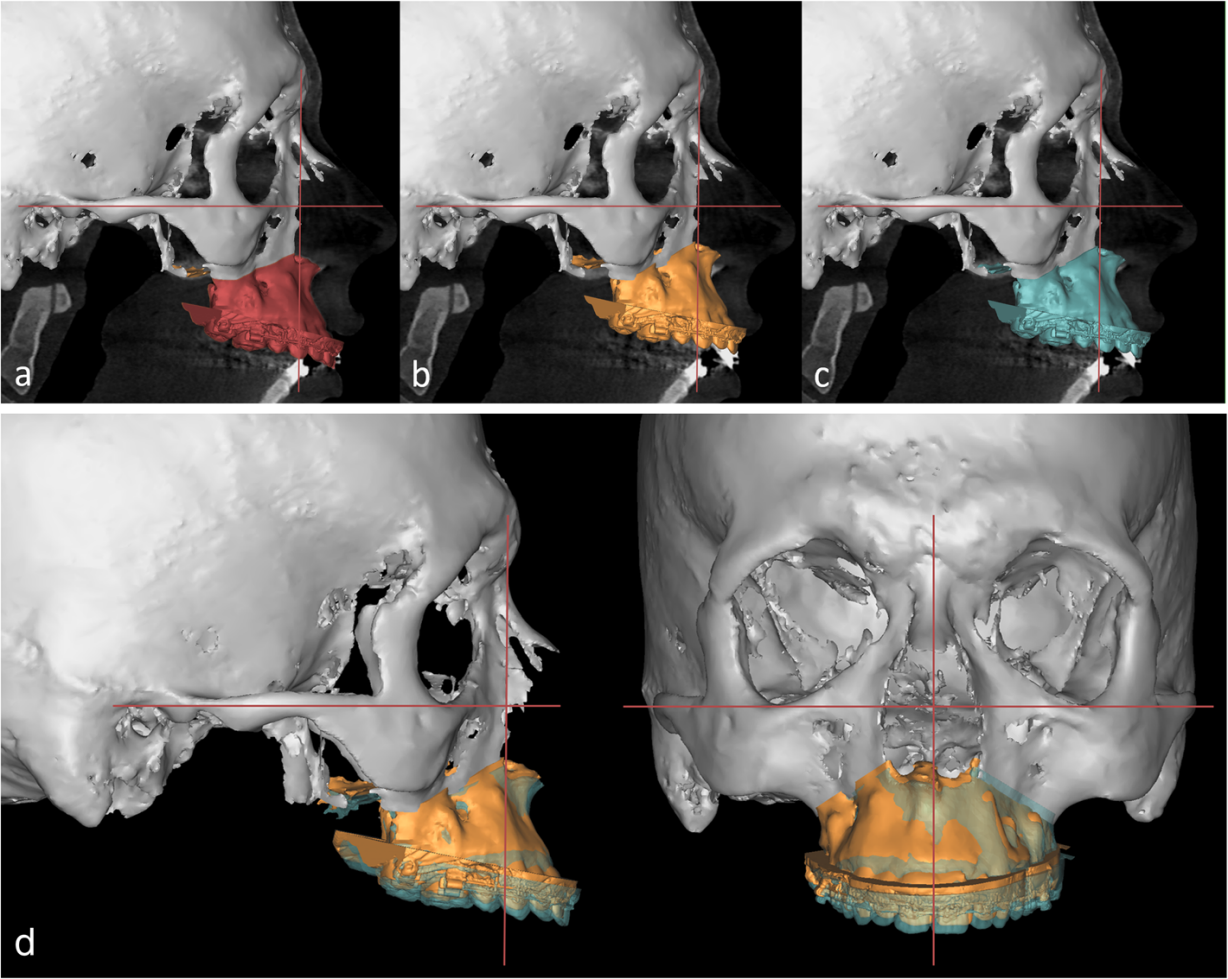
Comparison of the preoperative, the planned and the postoperatively achieved maxillary position: a Preoperative CBCT scan with the maxilla in the preoperative position. b Preoperative CBCT scan with the maxilla in the planned position. c Preoperative CBCT scan with the maxilla in the postoperatively achieved position. d The superimposition of the planned (yellow) and the achieved (green) maxillary position demonstrates the commonly observed underimpaction of the maxilla in vertical direction and a mild deviation of the midline in transversal direction
